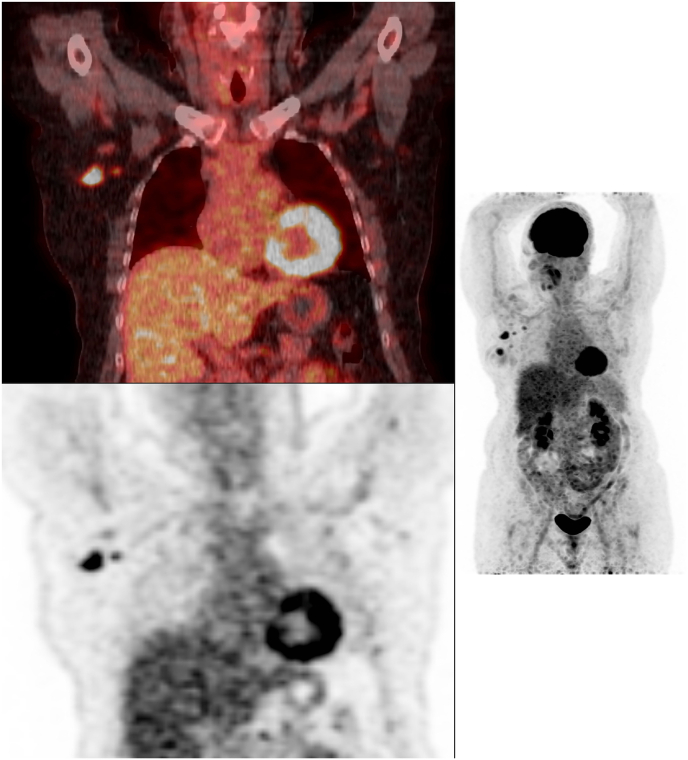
Baseline ^18^F-FDG PET/CT images of a RISAS patient with advanced baseline axillary disease (5 hypermetabolic ALNMs) with axillary residual disease at surgical staging. *FDG, fluorodeoxyglucose; ALNM, axillary lymph node metastases*.
